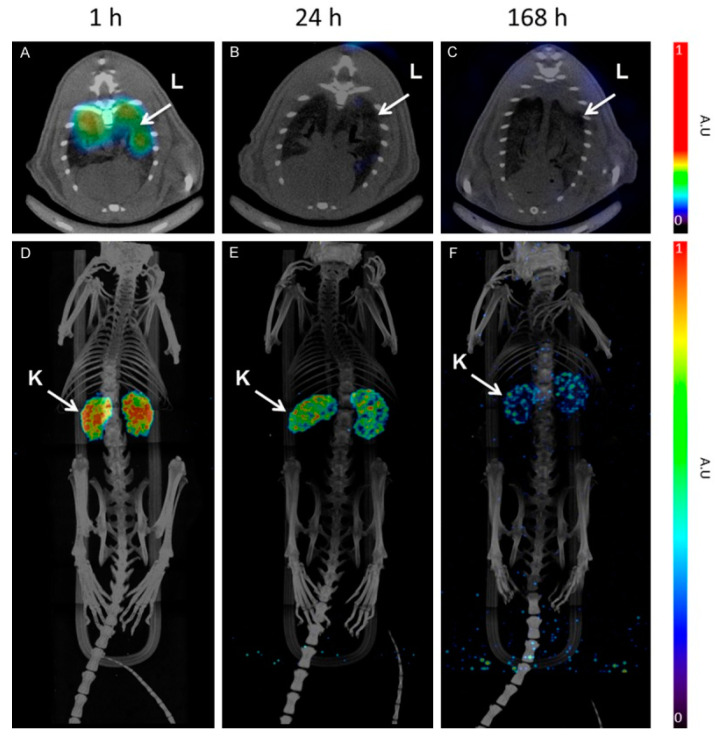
SPECT/CT images of [177Lu]-DO3A-VS-Cys40-Exendin-4 at 1, 4, and 168 h. Lungs are visible 1 h p.i. and show fast clearance (A–C). MIP images (D–F) of whole body showing mainly kidney uptake [47].
